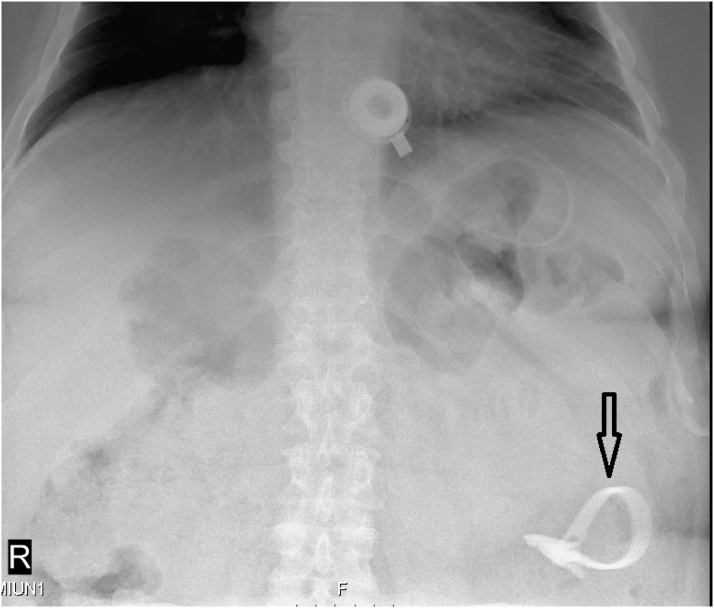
Plain abdominal X-ray showing gastric band localized to the left lower quadrant (arrow).
